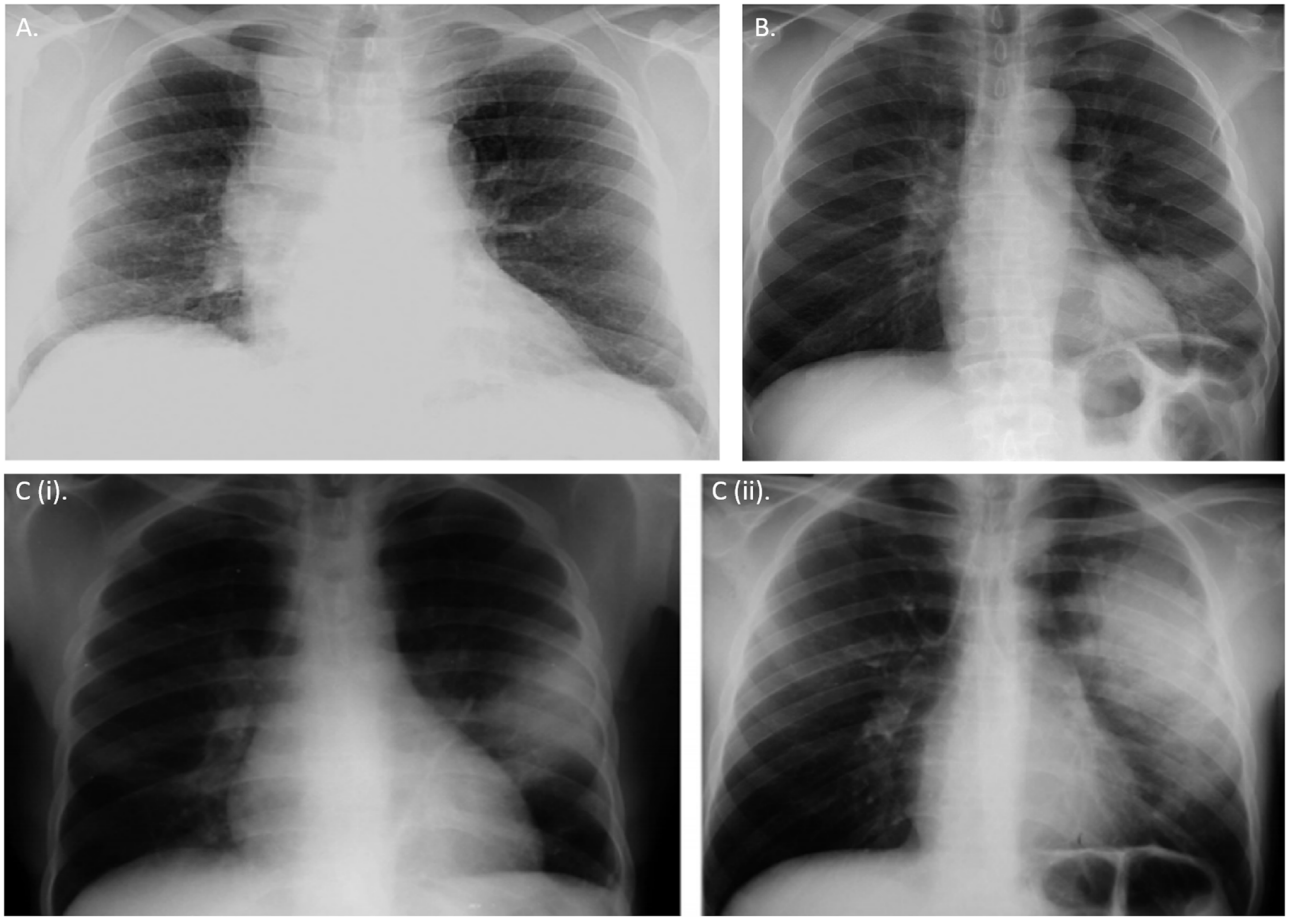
Chest radiographic appearances of tuberculosis in HIV. (A) Right hilar lymphadenopathy and bronchial markings in a primary pulmonary tuberculosis-like presentation in a HIV-infected young adult (B) Left lower lobe consolidation due to *Streptococcus pneumonia* infection in pre-existing tuberculosis and HIV coinfections (Ci,ii)
